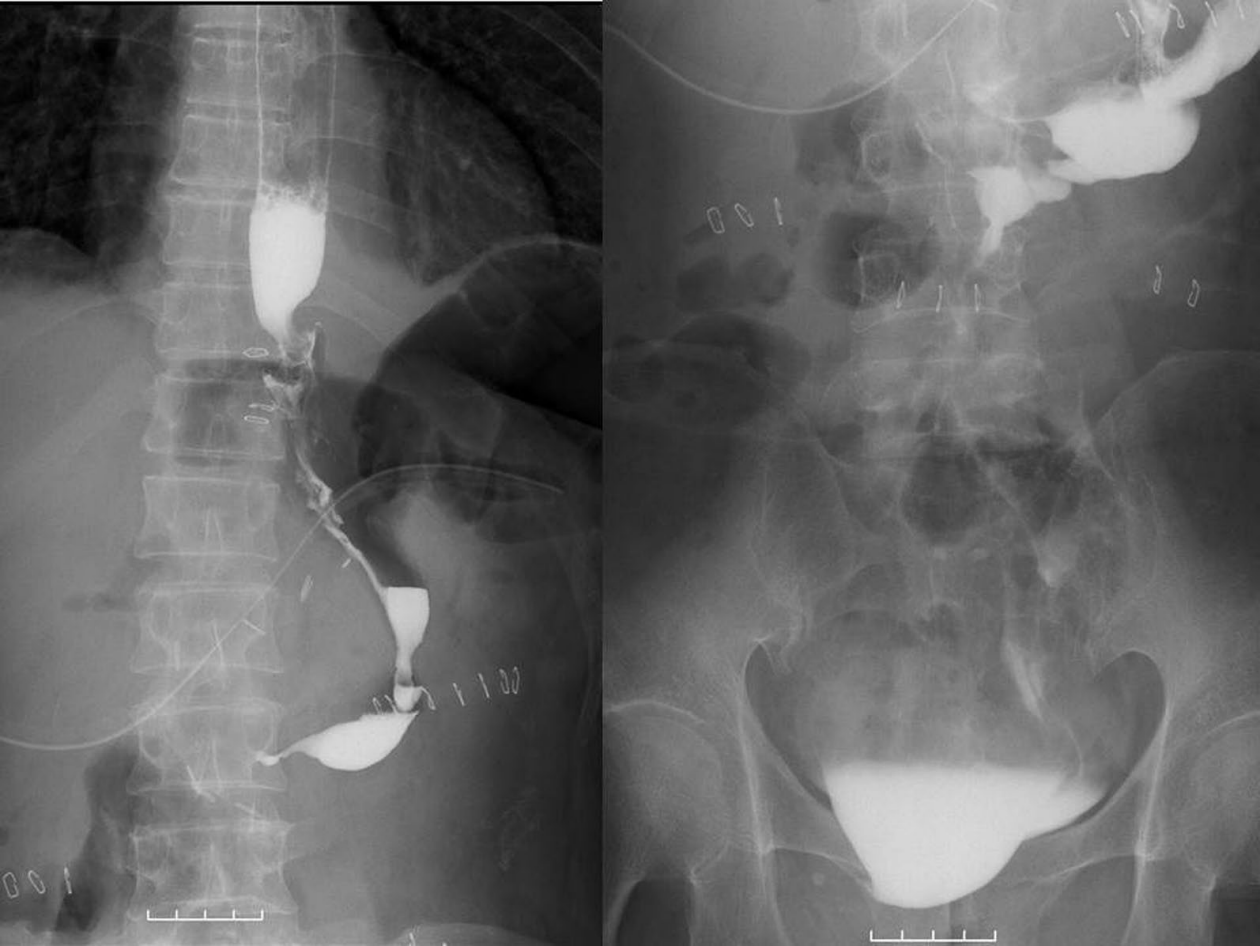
Oral contrast radiography. Gastrografin^®^ collections were evident, suggesting dehiscence
